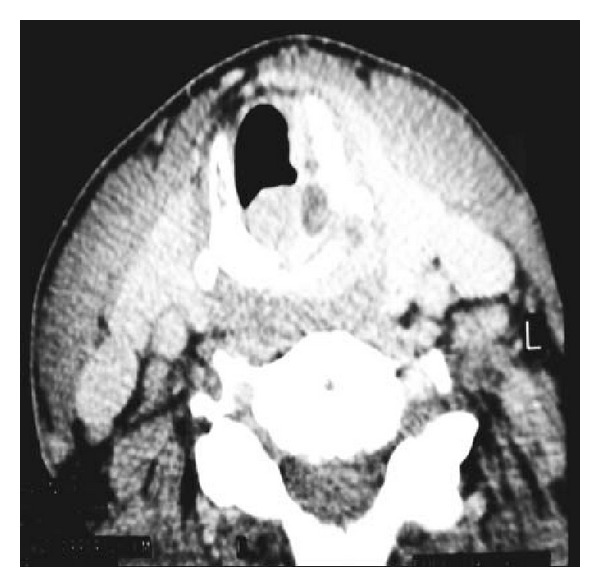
CT of neck. Invading mass of the larynx with subglottic and intralaryngeal extension.
